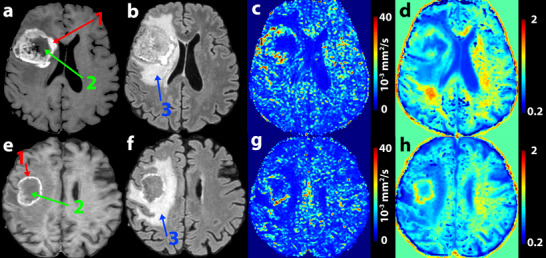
Example of two patients from the decision tree test cohort. The top row shows a patient (male, 67 years old) with a high‐grade glioma grade IV (HGG), and the bottom row shows a patient (male, 73 years old) with a colorectal carcinoma metastasis. On the post‐contrast T1‐weighted images (a and e), the enhancing (red arrow, Label 1) and non‐enhancing (green arrow, Label 2) tumor portions are visible. On the fluid‐attenuated inversion recovery (FLAIR) images (b and f), the edematous region (blue arrow, Label 3) can be seen. Only the multi‐*b* value maps that are used in the decision tree are displayed: pseudo‐diffusion (*D) (c and g) and diffusion kurtosis (*K*) (d and h). The median values are as follows: median *D in edema (10^−6^ mm^2^/s)—HGG = 4938, metastasis = 4544; median *K* in enhancing part—HGG = 0.822, metastasis = 0.927. Values used for decision tree evaluation include as follows: 95th percentile of *K* in enhancing region—HGG = 1.1103, metastasis = 1.3432; histogram kurtosis of *D in edema—HGG = 15.1931, metastasis = 34.4859; volume (cm^3^) of non‐enhancing tumor part—HGG = 13.246, metastasis = 12.221; fifth percentile of *K* in enhancing region—HGG = 0.5903, metastasis = 0.6538.
